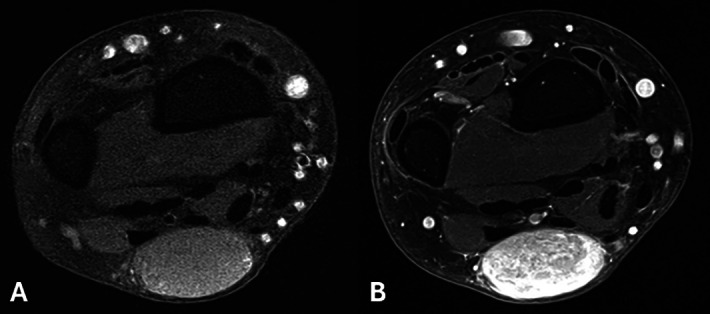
MRI. T1-WI before and after gadolinium contrast shows marked enhancement.
